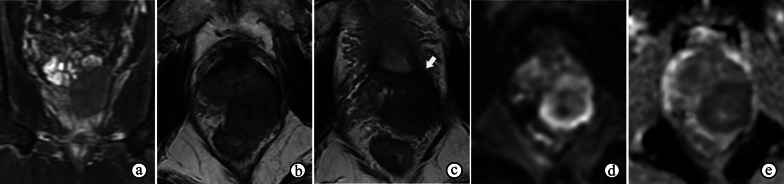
Local recurrence of urothelial carcinoma of the prostate in a 63-year-old man 1 year after completing chemotherapy. a–c Coronal fat-suppressed T2- (a) and axial T2WI (b, c) show an inhomogeneously isointense mass primarily located in the left lobe of the prostate and left seminal vesicle, invading a corner of the bladder (arrow on c). d, e DWI at a b-value of 1400 s/mm^2^ and ADC maps show restricted diffusion in the periphery of the mass
